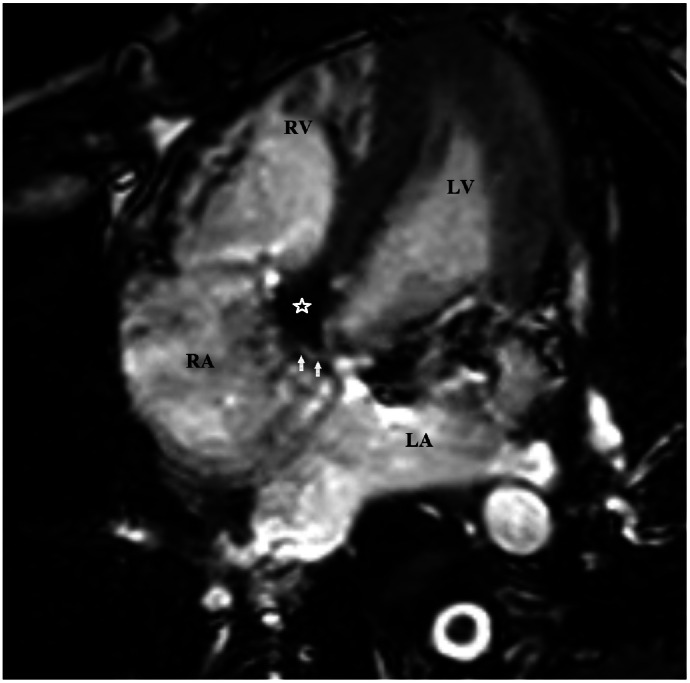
Cine MRI in a 4-chamber view after the first transcatheter closure in the first patient. residual left-to-right shunt (white arrow) is still visible alongside the implanted LifeTech 20 × 22 mm occluder device (*). This residual jet was eliminated after a second procedure.
